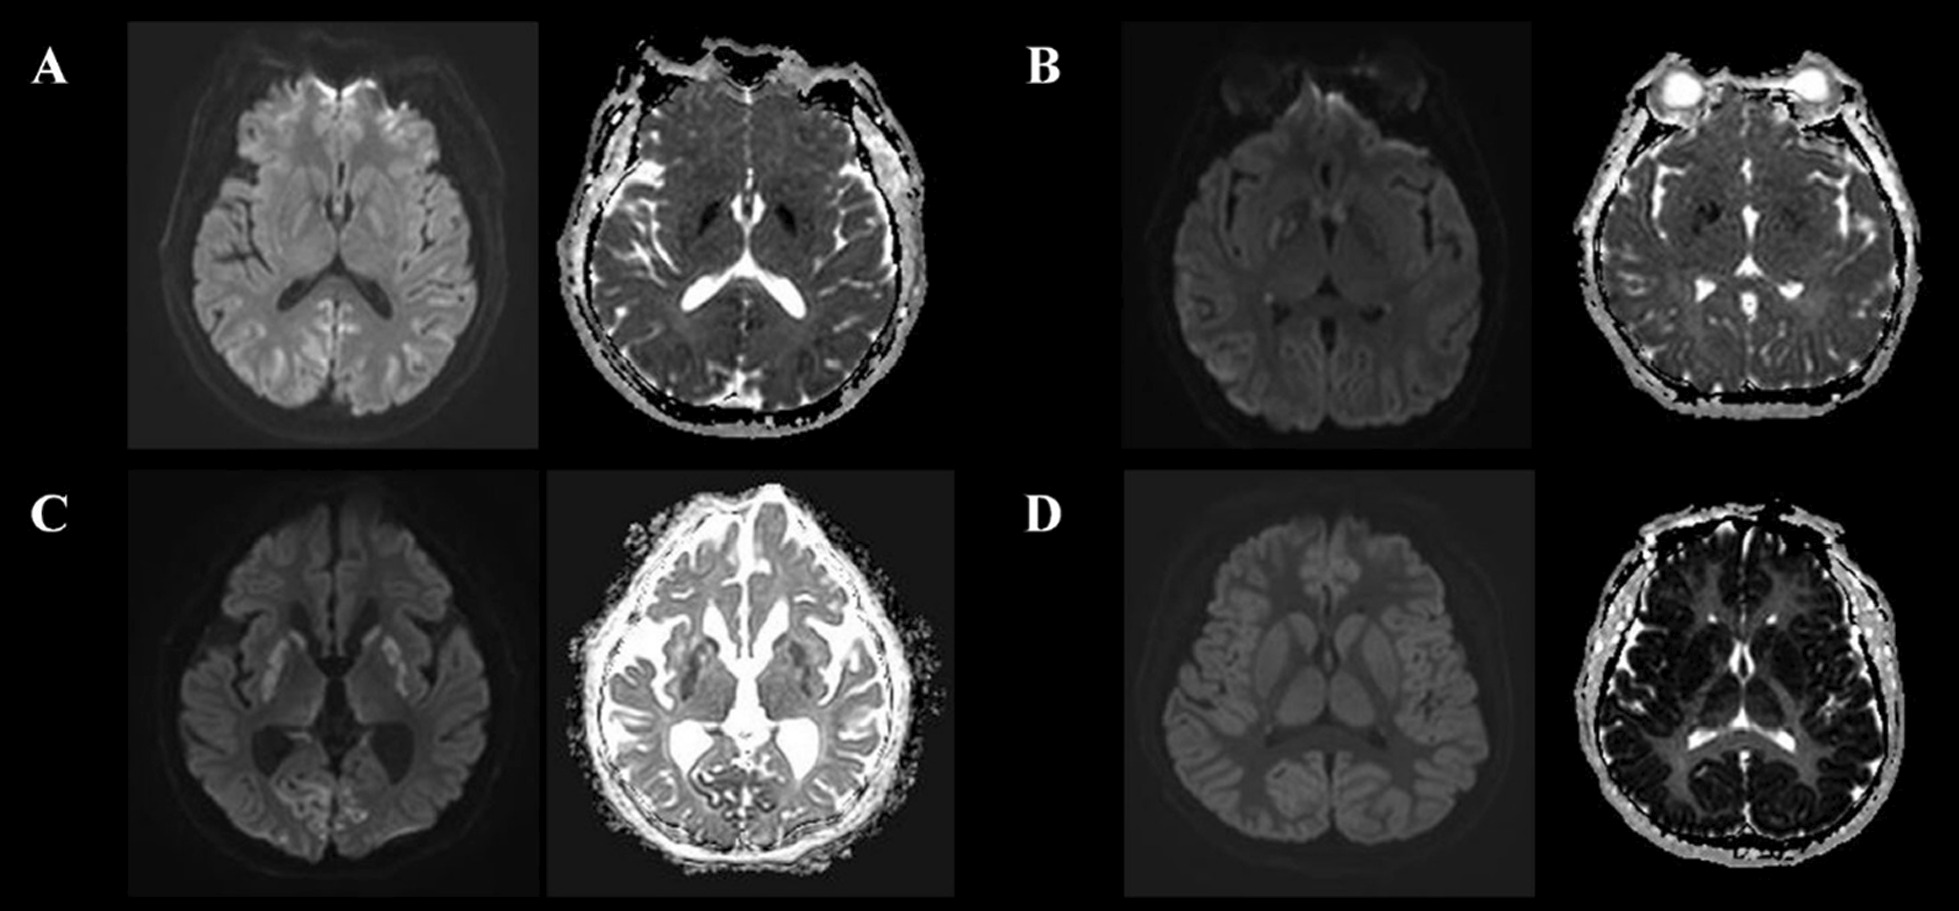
Various pattern of ultra-early DW-MRI and ADC of OHCA survivals. A. A 70-years old male. Gyriform restrictive diffusion in occipital cortex. B. A 31-years old female. Gyriform and regional restrictive diffusion in occipital cortex, temporal cortex, and deep gray matter, respectively. C. A 74-years old male. Gyriform and regional restrictive diffusion in occipital cortex and deep gray matter, respectively. D. A 58-years old male. Extensive gyriform resticted diffusion in all gray matter. *Abbreviations:*
